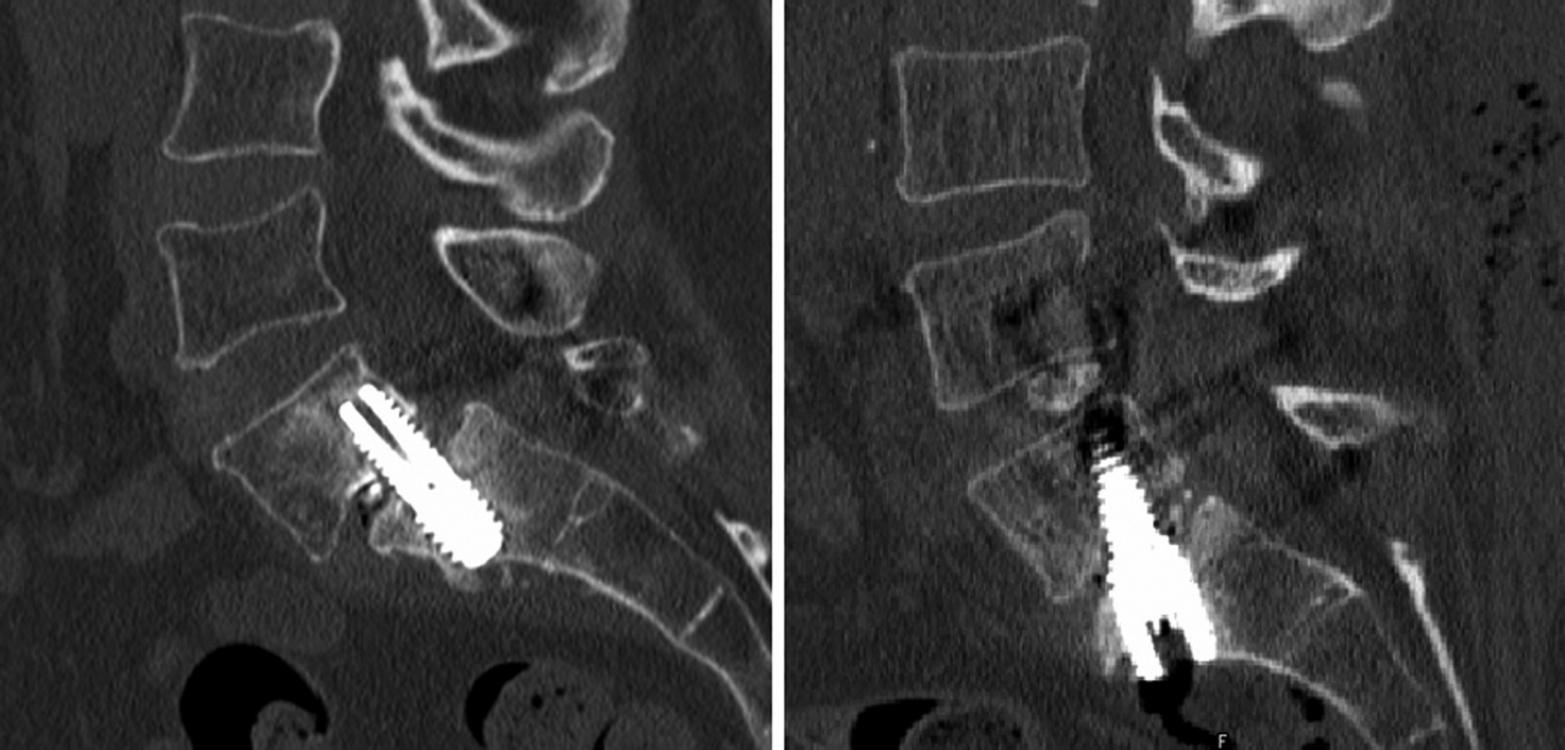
Computed tomographic midline sagittal images of the lumbosacral spine demonstrate postoperative spinal alignment for L5-S1 (case 1, left) and L4-5, L5-S1 (case 3, right) constructs.
